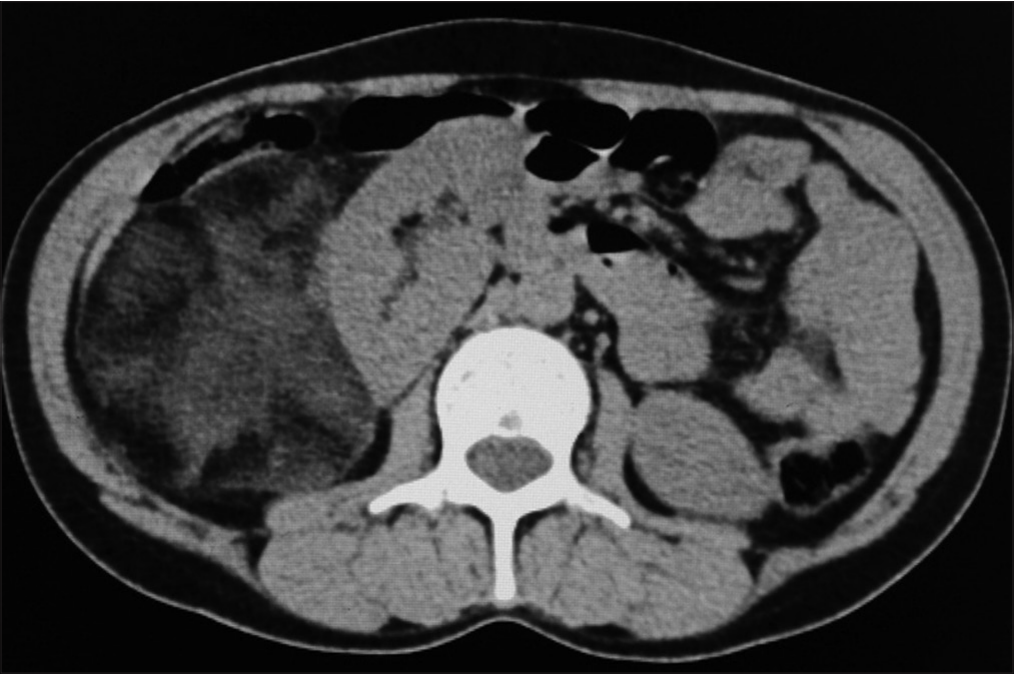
Noncontrast transverse CT image showing a large well-delineated fatty mass with some dense attenuation areas inside on the lateral aspect of right kidney which is displaced medially
